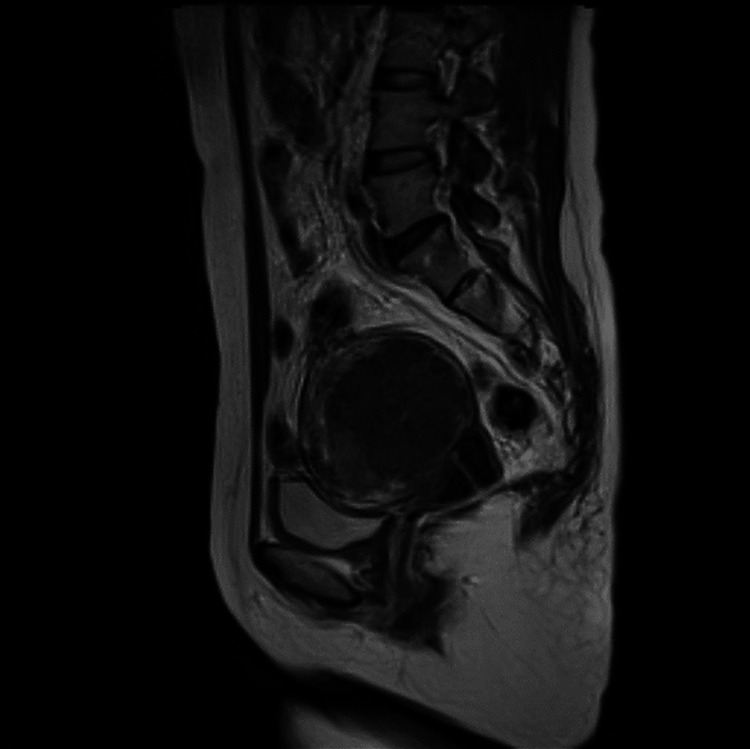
MRI finding at first presentation A well-defined ~7 cm leiomyoma was identified in the uterine fundus on the T2-weighted image.
